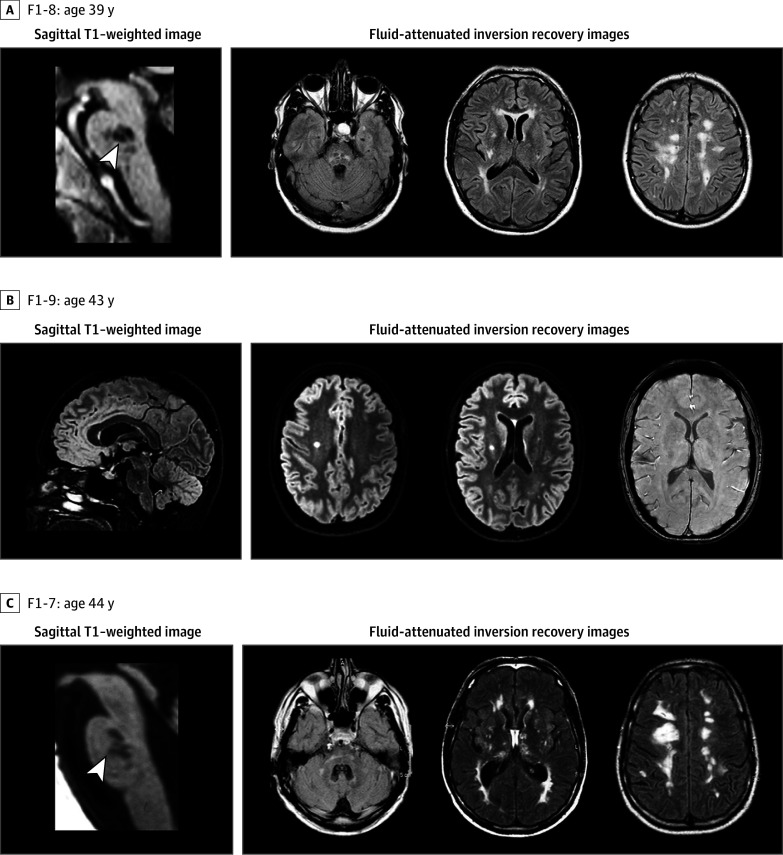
Magnetic Resonance Imaging Data of Patients From Family F1 The first column presents sagittal T1–weighted images. Fluid-attenuated inversion recovery images are presented in columns 2 to 4. All patients present pontine infarcts and a vascular leukoencephalopathy associated with hemispheric lacunes.
